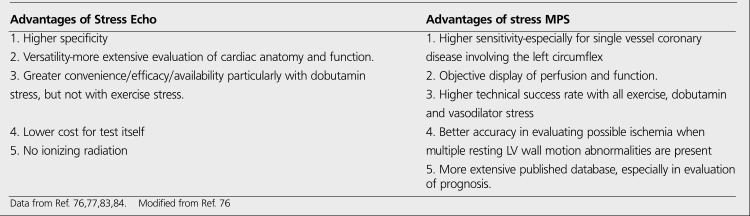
Comparative advantages of stress echocardiography and stress MPS in diagnosis of CHD
